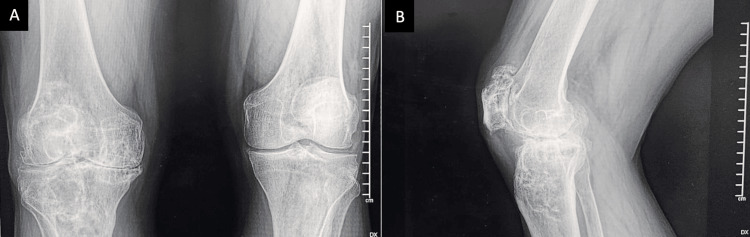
Radiographic images of the right knee A: anteroposterior; B: lateral projections show a bilateral reduction in joint space with varus deformity. Marginal lytic bone lesions are noted at the subchondral level of the proximal tibia and distal femur, exhibiting well-defined sclerotic edges and no periosteal reaction. At the patellar level, there is a reduction in joint space along with marginal osteophytes present at the upper pole.
